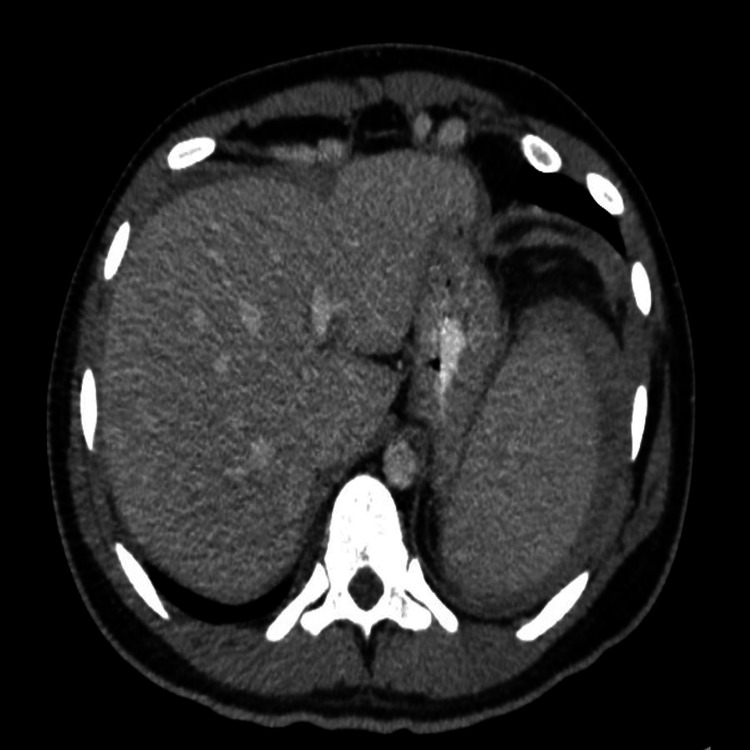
CT scan of the abdomen with intravenous contrast in the transverse plane
